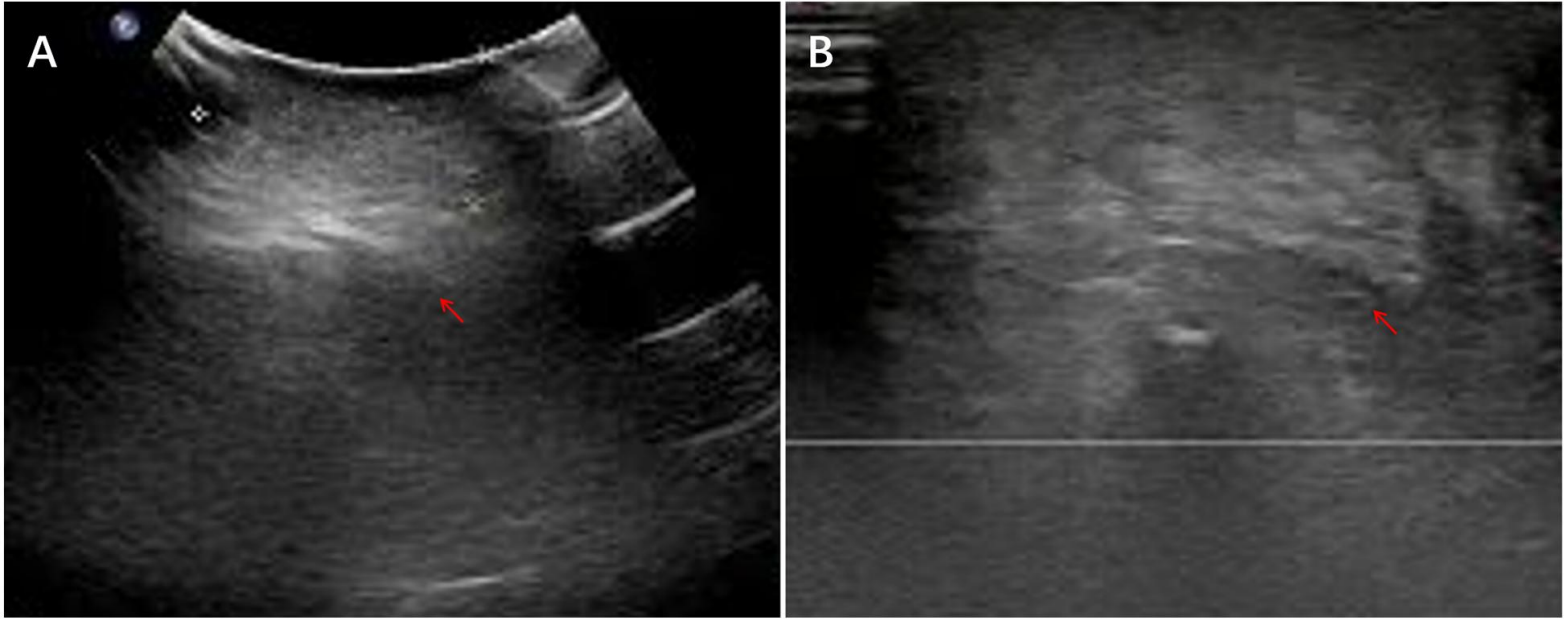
Ultrasound images of the left gluteal region IMT. (A) A hypoechoic solid subcutaneous mass with heterogeneous internal echotexture. (B) The mass shows poorly defined margins and an irregular morphology.
